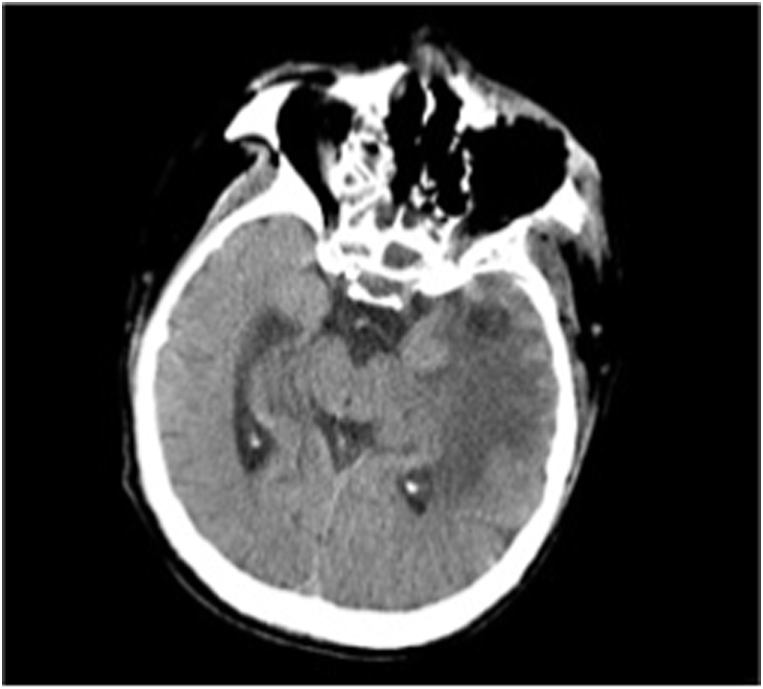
Imaging of the Patient A. Computed tomography scan of the head showed pansinusitis and left orbital necrosis B. Contrast-enhanced computed tomography scan of the head showed cerebral abscess and edema in the left temporal region.
